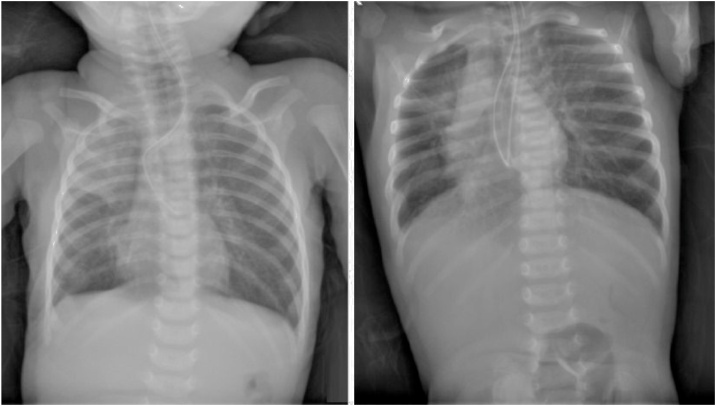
Plain chest x-ray taken on the 16th day of life shows rigid tube with tip at T8 vertebra. Part of the tube is bent with irregular orientation inside the wide esophagus. Repeat x-ray on the same day also shows coiling of the tube around the same level.
